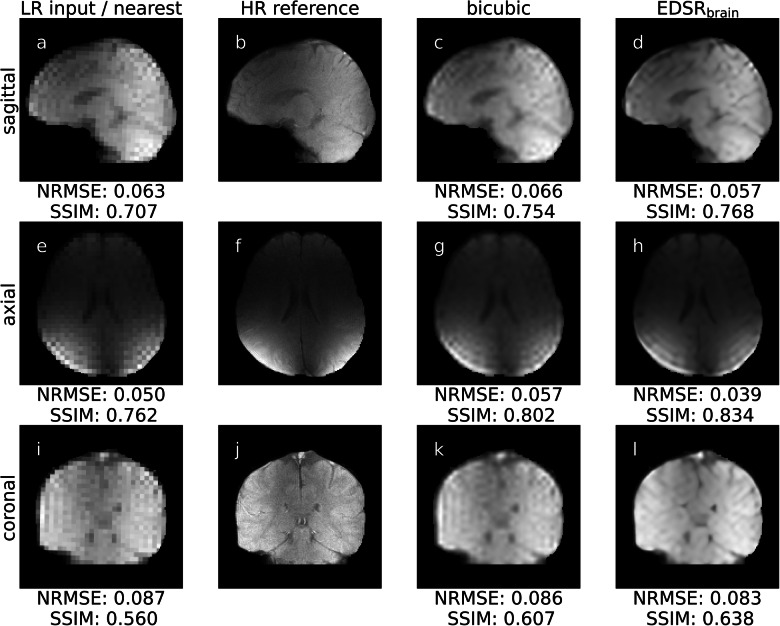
Super-resolution on orthogonal single-image brain MRIs. These MRIs were acquired on a prototype 1.0 T MRI-linac. Quick-to-acquire low spatial resolution acquisitions (LR input / nearest [a], [e], [i]) were input to super-resolution technique bicubic interpolation [c], [g], [k] and EDSR_brain_ [d], [h], [l]. These super-resolution images were then compared to a long-to-acquire high spatial resolution (HR reference [b], [f], [j]) through normalised root mean-square-error (NRMSE) and structural similarity (SSIM). Additionally, the low spatial resolution acquisitions had nearest neighbour applied to allow for quantitative analysis with the high spatial resolution reference. All images scaled between 0 and 1. LR low resolution, EDSR enhanced deep super-resolution, HR high resolution.
